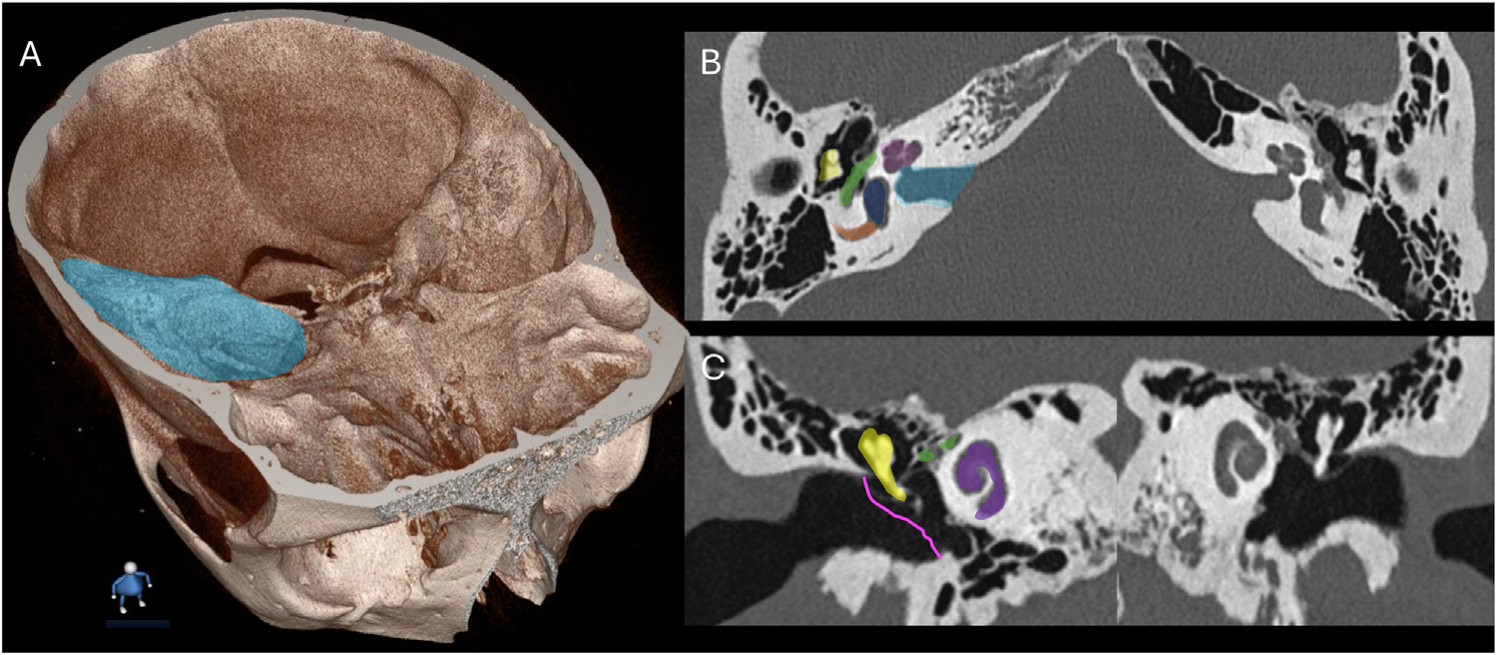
3D computed tomography (CT) of the human temporal bone and inner ear. A) CT of a human skull with the temporal bone highlighted in blue. Corresponding B) axial and C) coronal CT images demonstrating normal temporal bone structures, color‐coded on the left side (patient's right side) for reference to the opposite side. Purple, cochlea. Yellow, ossicles. Green, facial nerve. Light blue, internal auditory canal. Dark blue, vestibule. Orange, posterior semicircular canal. Pink, tympanic membrane.
